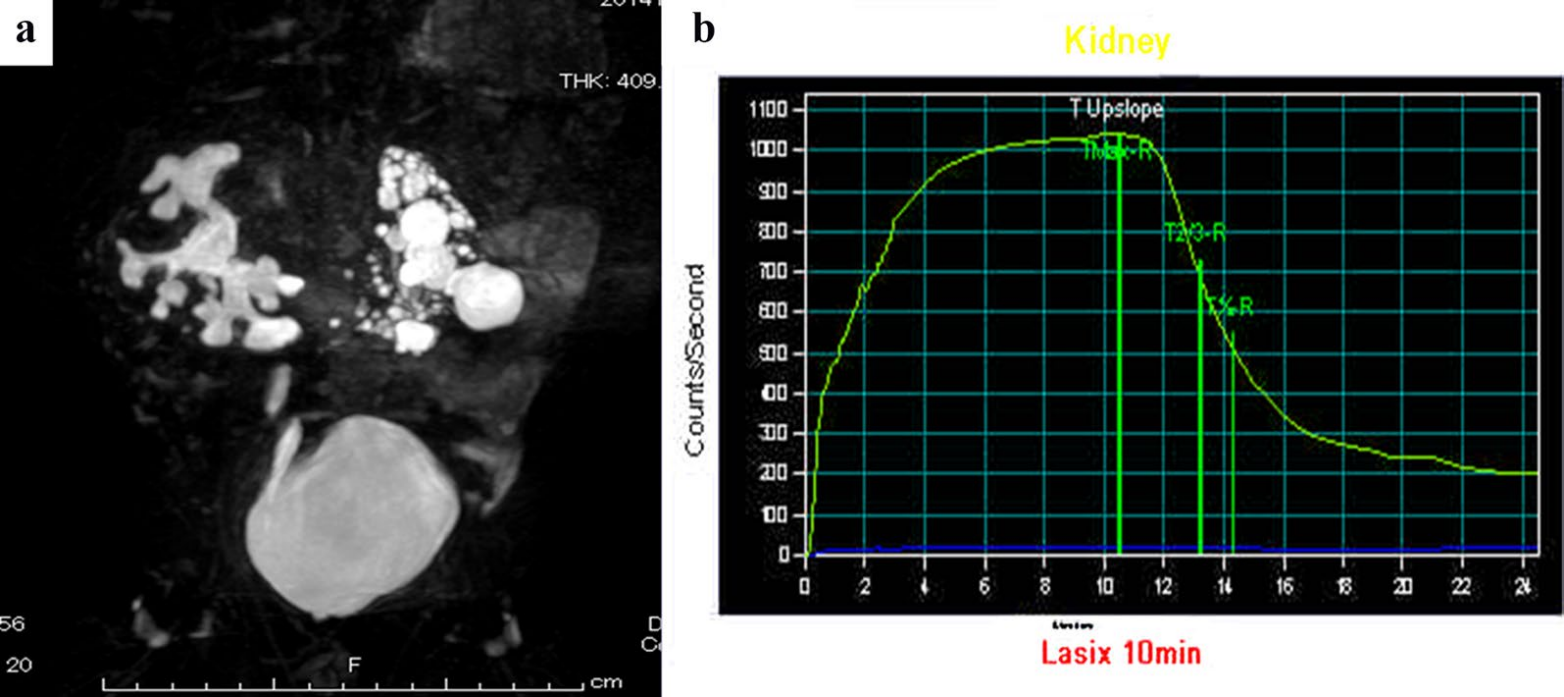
Postoperative MRU and renal scintigraphy findings. a MRU, b scintigraphy
